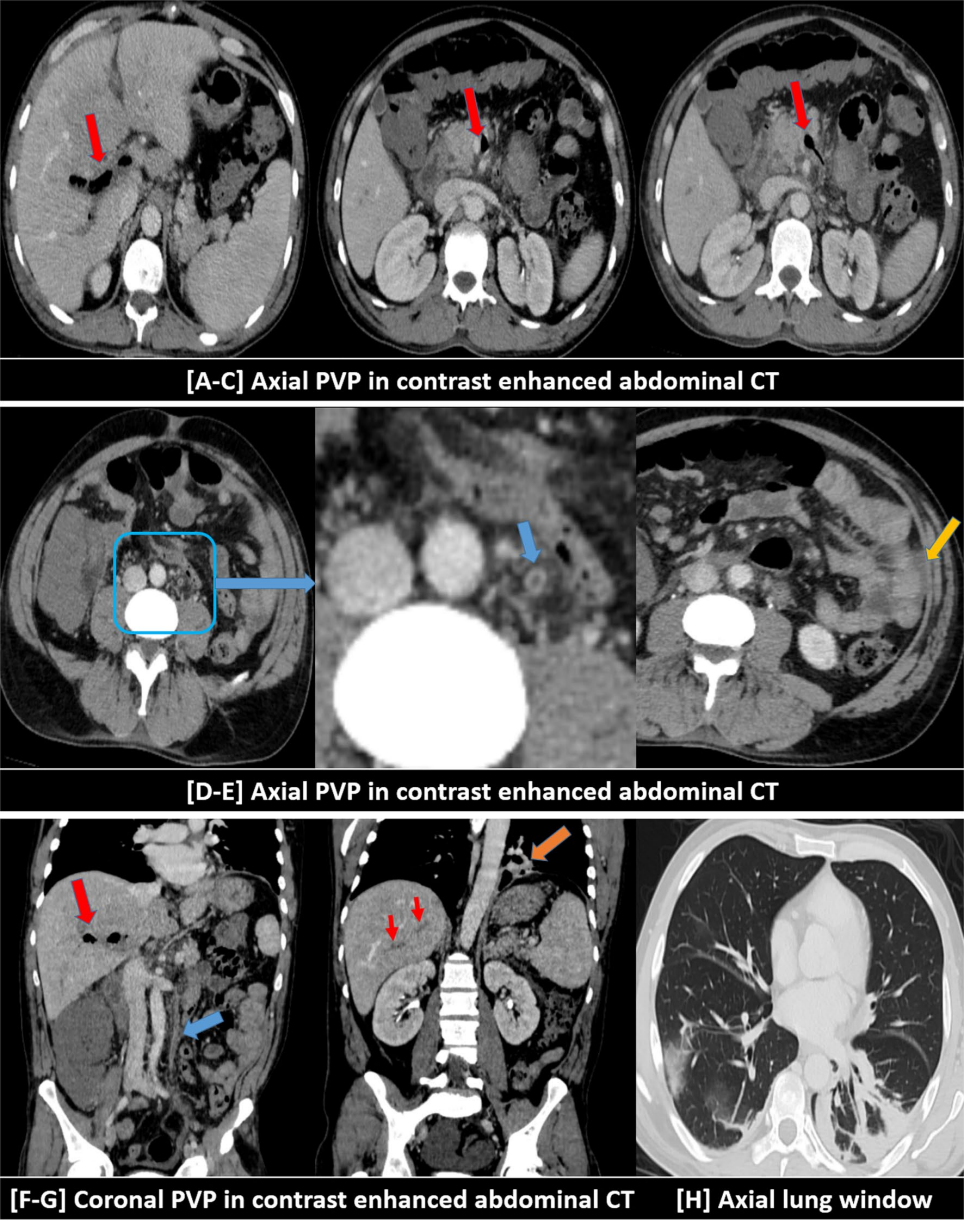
A 63-years-old male patient was proved for COVID-19 infection. He presented to the emergency unit during the second week of infection with severe abdominal pain. Mesenteric CT arteriography and venography were performed without oral preparation. [A–C] Axial images of abdominal CT at the venous phase revealed thrombosis of the portal venous system till the porto-mesenteric confluence and proximal part of the superior mesenteric vein (SMV) with intra-luminal air (red arrows). [D] Axial image of abdominal CT at the venous phase with zoomed images revealed thrombosis of the inferior mesenteric vein (IMV) (blue arrow). [E] Axial CT image showed mild mural edematous changes of the jujenal bowel loops with mild fluid collection in between denoting ischemic changes (yellow arrow). [F, G] Coronal images of abdominal CT at the venous phase revealed thrombosis of the portal vein with internal air (red arrows), thrombosis of the inferior mesenteric vein (blue arrow), and left basal pulmonary consolidative patch (orange arrow). [H] Axial image of the basal lung zones (lung window) revealed a right basal sub-pleural ground-glass patch and left basal consolidative atelectatic patch (typical for COVID19 infection)
